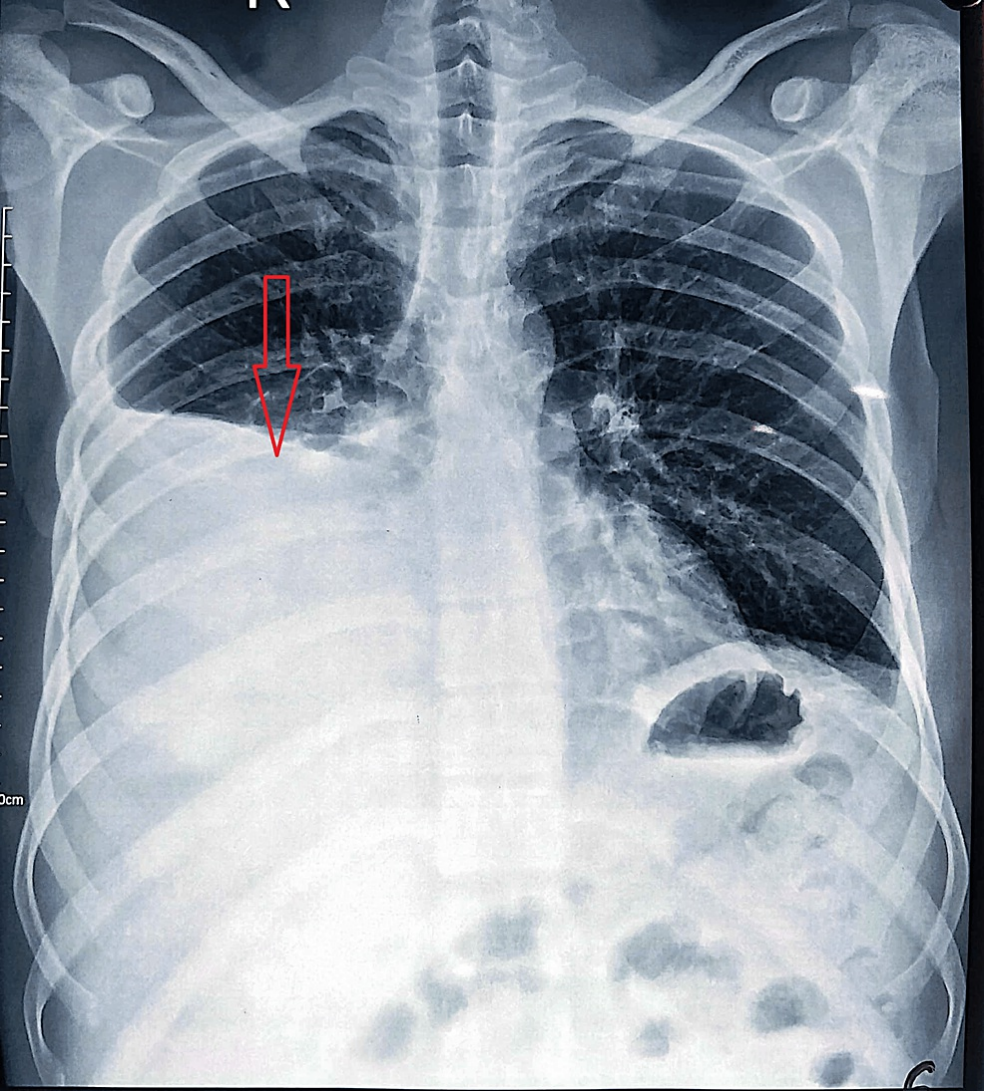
Chest X-ray showing right-sided pleural effusion
